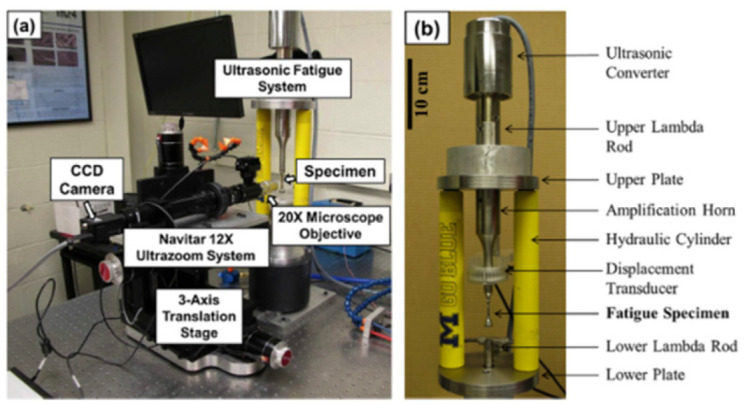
Photos of the Navitar 12X Ultrazoom optical system and ultrasonic testing system [39].
